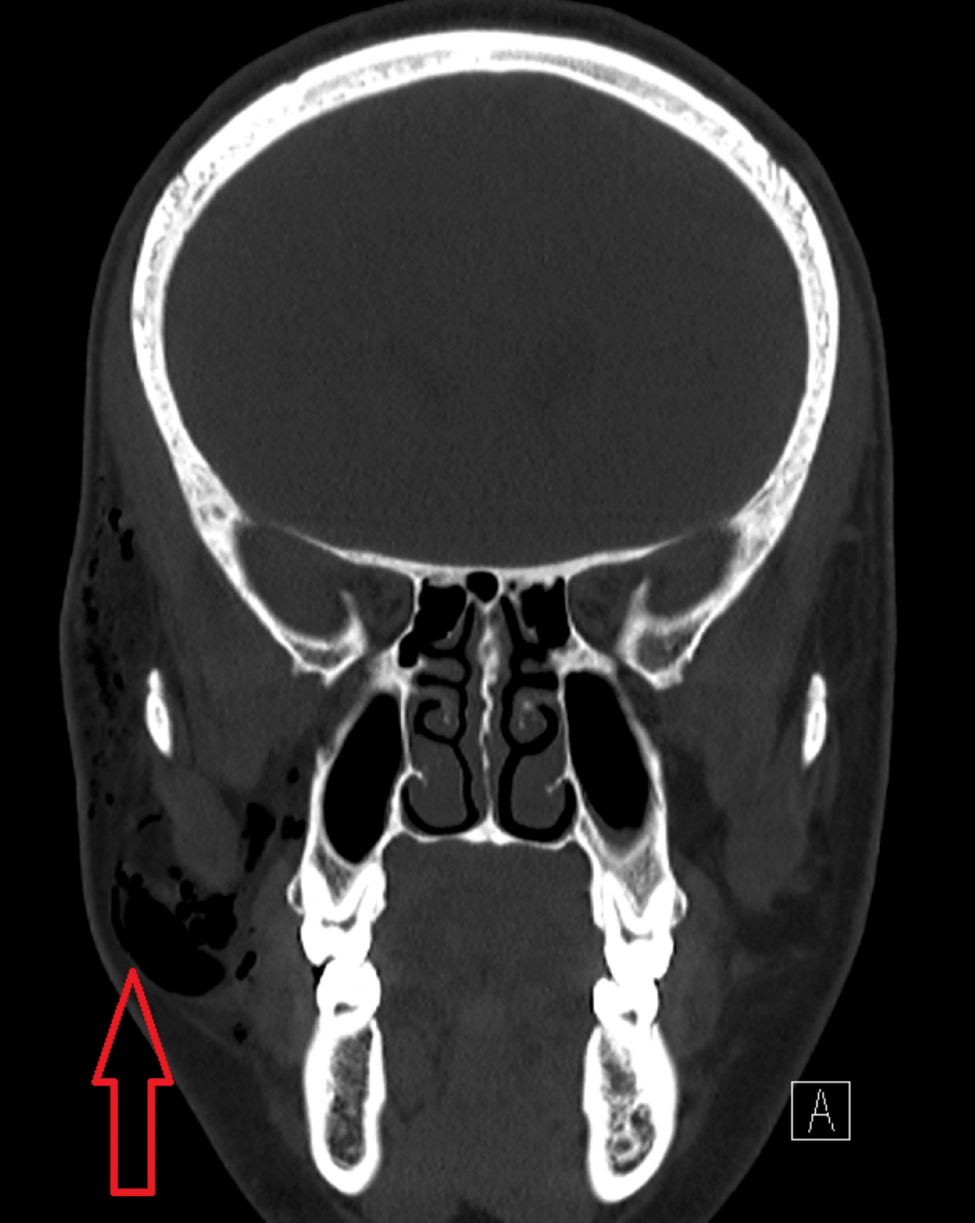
Coronal CT view showing the extent of right orbital subcutaneous emphysema as pointed out by the red arrow
